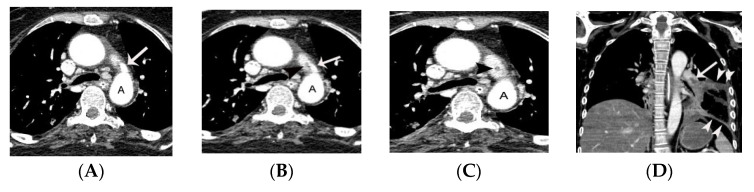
Septic pulmonary infarction caused by embolization of vegetation or endarteritis of a PDA in a 42-year-old female with history of glioblastoma multiforme. Small conically shaped PDA (arrow) is noted on contiguous axial CT images (A–C). Note small nodular lesion (arrowhead on C) at the pulmonic end of the PDA, suggesting a vegetation or endarteritis. This finding was confirmed by subsequent echocardiography. Septic embolus is noted in the pulmonary artery in the left lower lobe (arrow on D) on a coronal CT image at the level of descending thoracic aorta. Septic pulmonary infarction with necrosis (arrowheads on D) is also demonstrated in the left lower lobe. This patient eventually died due to septic shock. A indicates aorta.
